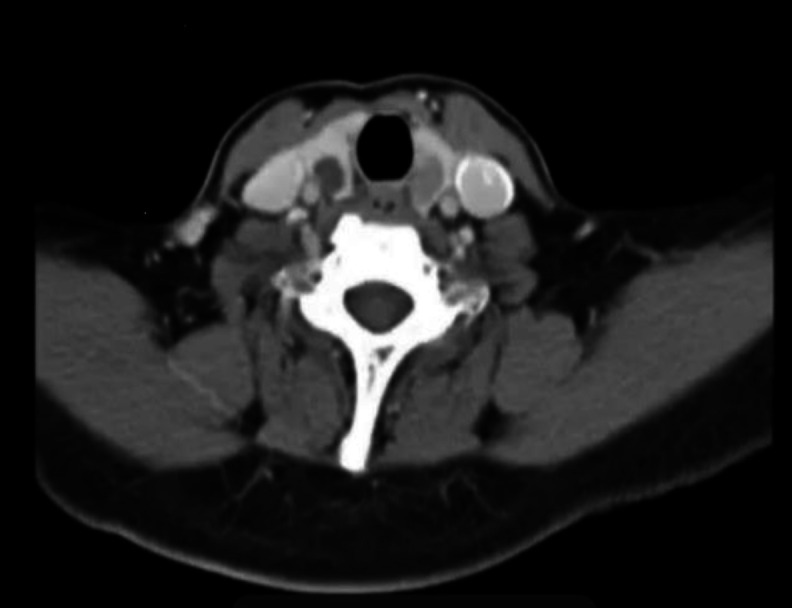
Axial CT image showing bilateral thyroid nodules
